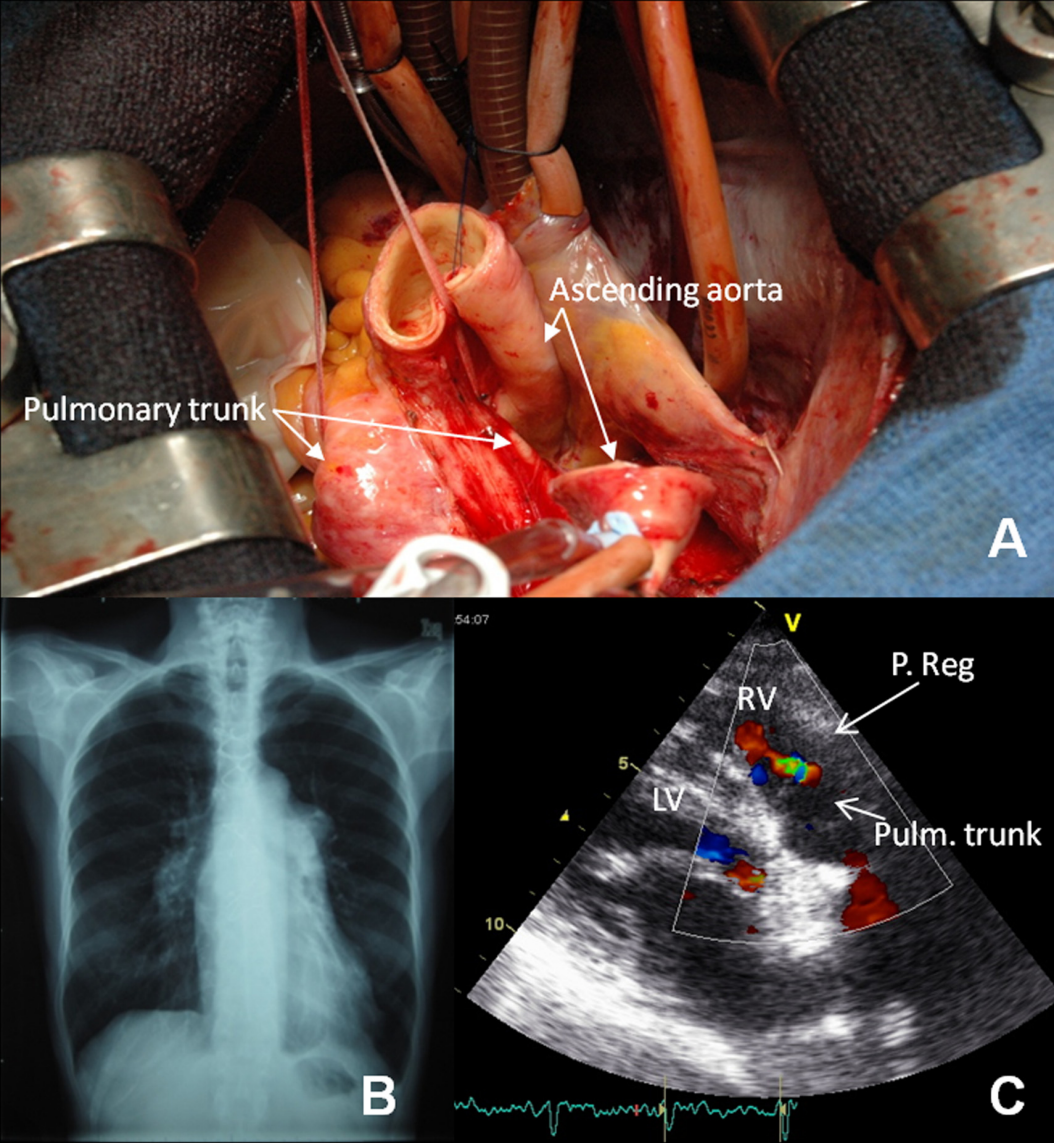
Surgical procedure and imaging exams after corrective surgery. A) Pulmonary artery aneurysm surgery: transverse aortotomy and visualization of the dilated pulmonary trunk. B) Post surgical chest radiograph (posteroanterior view) showing a narrower cardiac silhouette, without visualization of the main pulmonary artery. C) Post surgical echocardiogram showing only mild pulmonary regurgitation.
